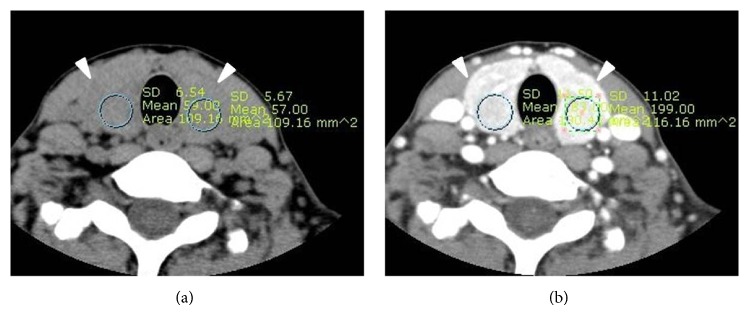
A measurement method of parenchymal Hounsfield unit (HU) by using regions of interest (ROI) (arrows) in nonenhanced (a) and contrast-enhanced (b) CT images.
